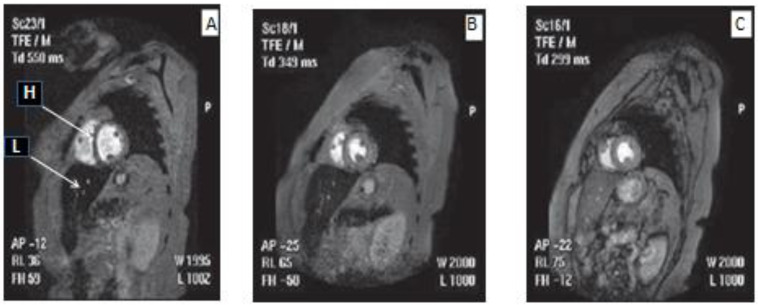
Clearing of excess iron from the heart and the liver of an iron-loaded thalassemia patient using the deferiprone and deferoxamine ICOC combination therapy protocol. The pictures show the magnetic resonance imaging (MRI) changes during the deferiprone/deferoxamine ICOC combination therapy. Short axis view of liver (L) and heart (H) of an iron-loaded male thalassemia patient (38 years, 69 Kg) at 5 months before the deferiprone/deferoxamine combination (Picture (A): Cardiac T2* was 14.5 ms and liver T2* 3.7 ms. Serum ferritin was 1626 μg/L, (estimated 1 month after the MRI scan); 9 months after the combination (Picture (B): Cardiac T2* was 17 ms and liver T2* 8.4 ms. Serum ferritin was 686 μg/L, (estimated 5 months after the MRI scan); and twenty and a half months after the combination, where normal iron levels have been achieved (Picture (C): Cardiac T2* was 20.7 ms and liver T2* 18 ms. Serum ferritin was 186 μg/L, (estimated 2.5 months after the MRI scan). ICOC: International Committee on Chelation T: signal intensity relaxation time. MRI: magnetic resonance imaging. Adapted with permission from reference [159].
